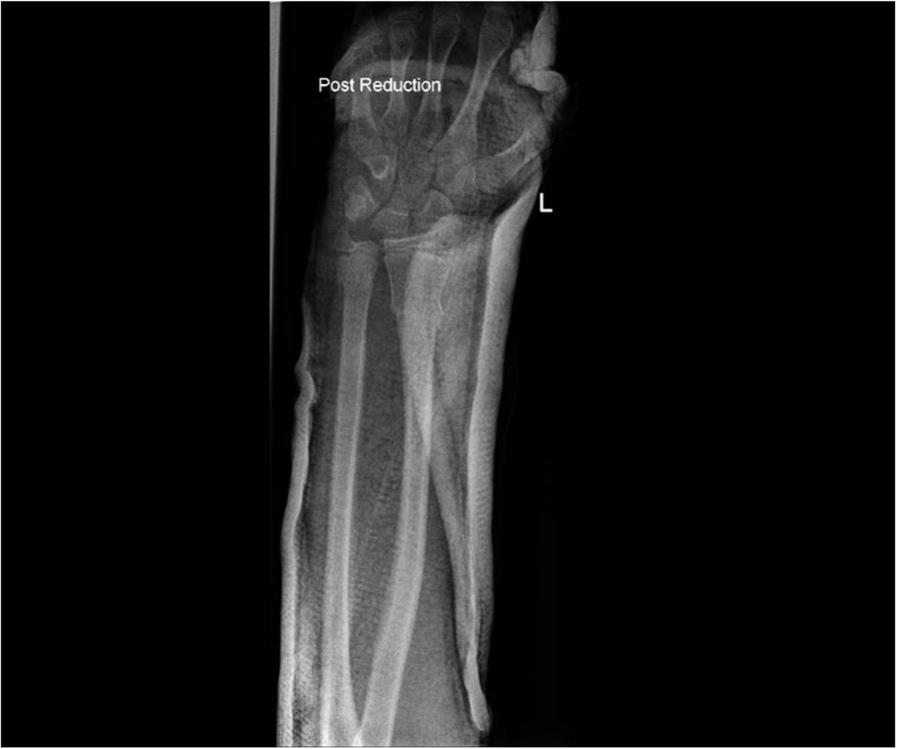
Pre- and post-manipulation radiographs of an 11-year-old boy with a left distal forearm fracture
